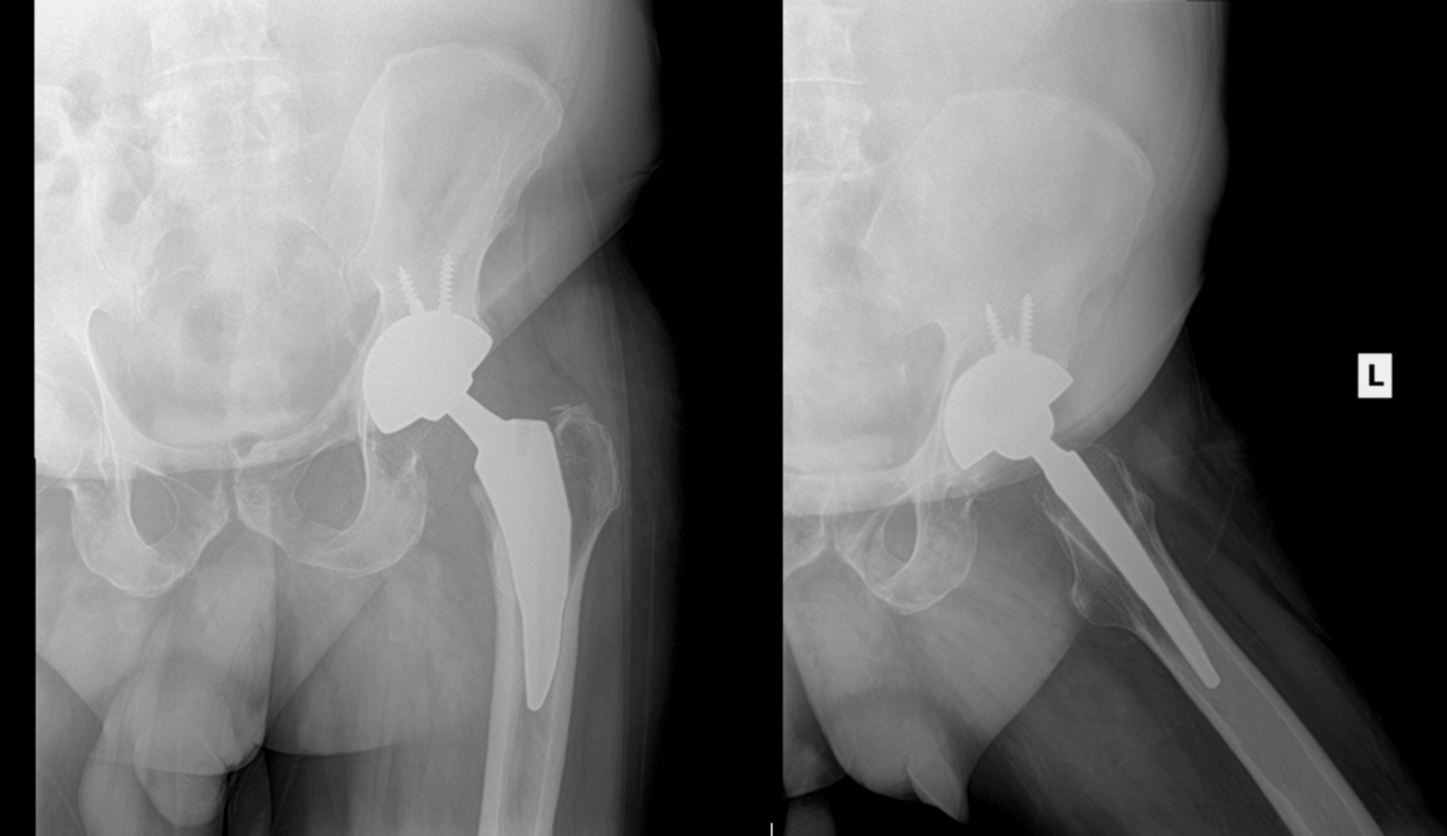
Anteroposterior and lateral X-ray of the left hip two weeks post-operatively demonstrating appropriate implant placement
